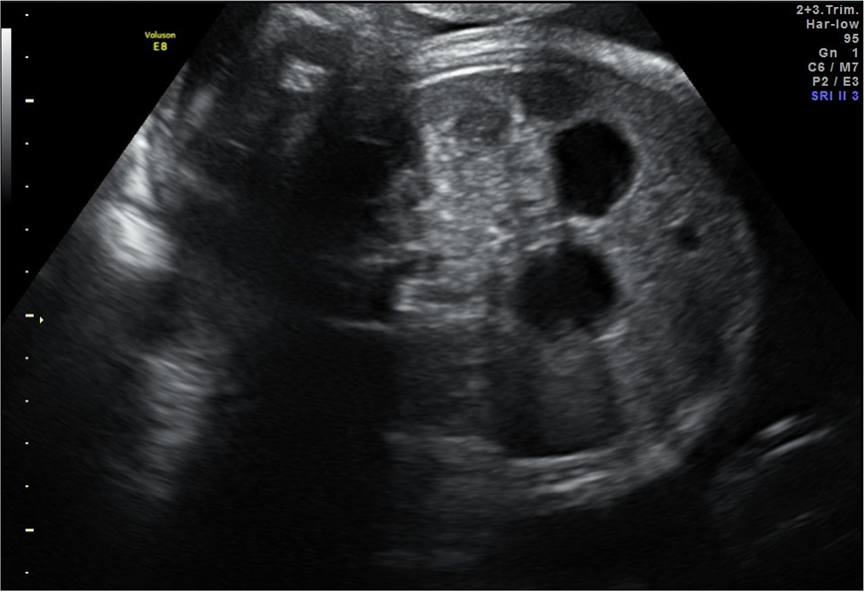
Antenatal ultrasound at 36 weeks gestation. Two fluid filled structures in the fetal abdomen: a ‘double bubble’ sign.
